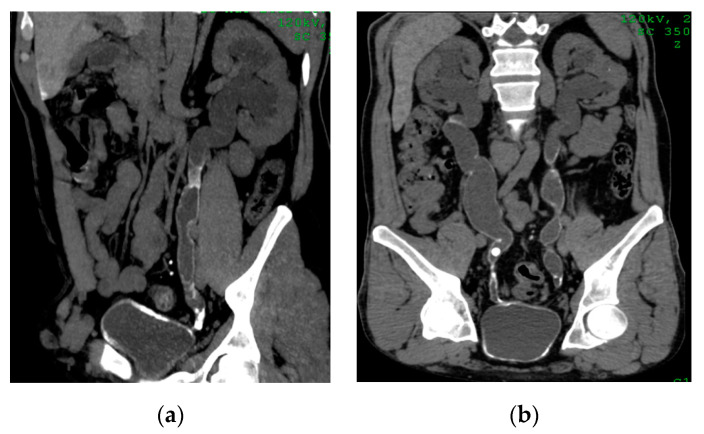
Urinary bilharziasis. (a,b) Coronal reformatted CT images without contrast showing diffuse mural calcifications of the bladder, and both ureters with bilateral hydroureteronephrosis and multiple bilateral ureteric strictures associated with secondary stone at right pelvic ureter.
